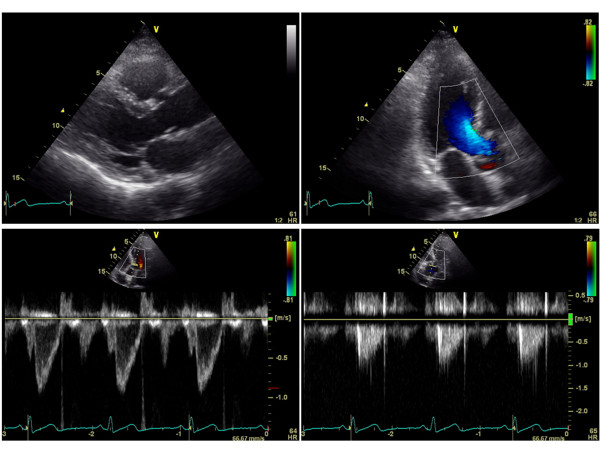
Two-dimentioal and Doppler echocardiographic images from a patient without CKD or CAD. CKD: chronic kidney disease; CAD: coronary artery disease.
